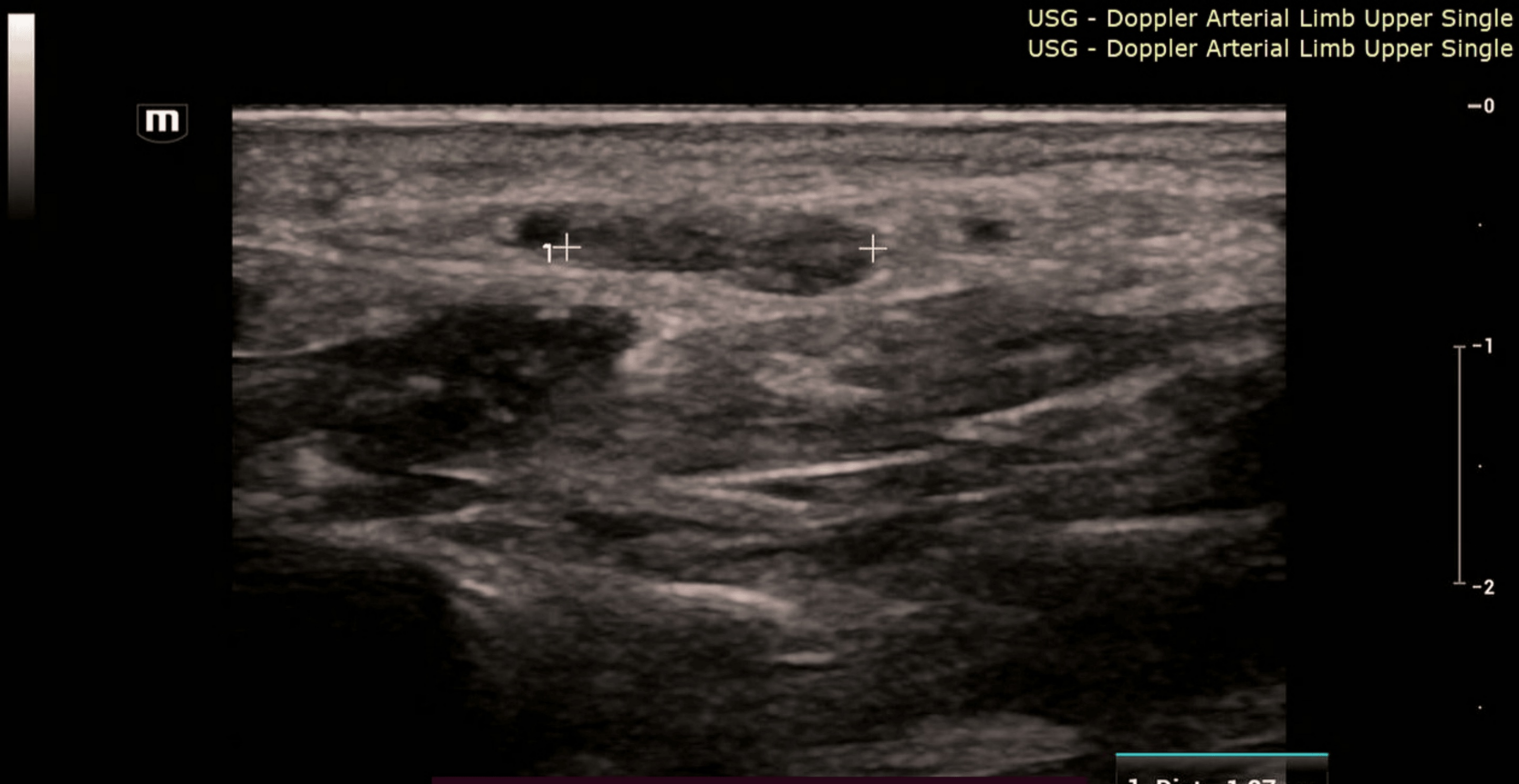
Arterial doppler of hand. Arterial Doppler revealed a short segment acute thrombus/embolus in the superficial palmar arch with absent distal flow signals, preserved proximal flow, and no significant proximal vessel stenosis.
